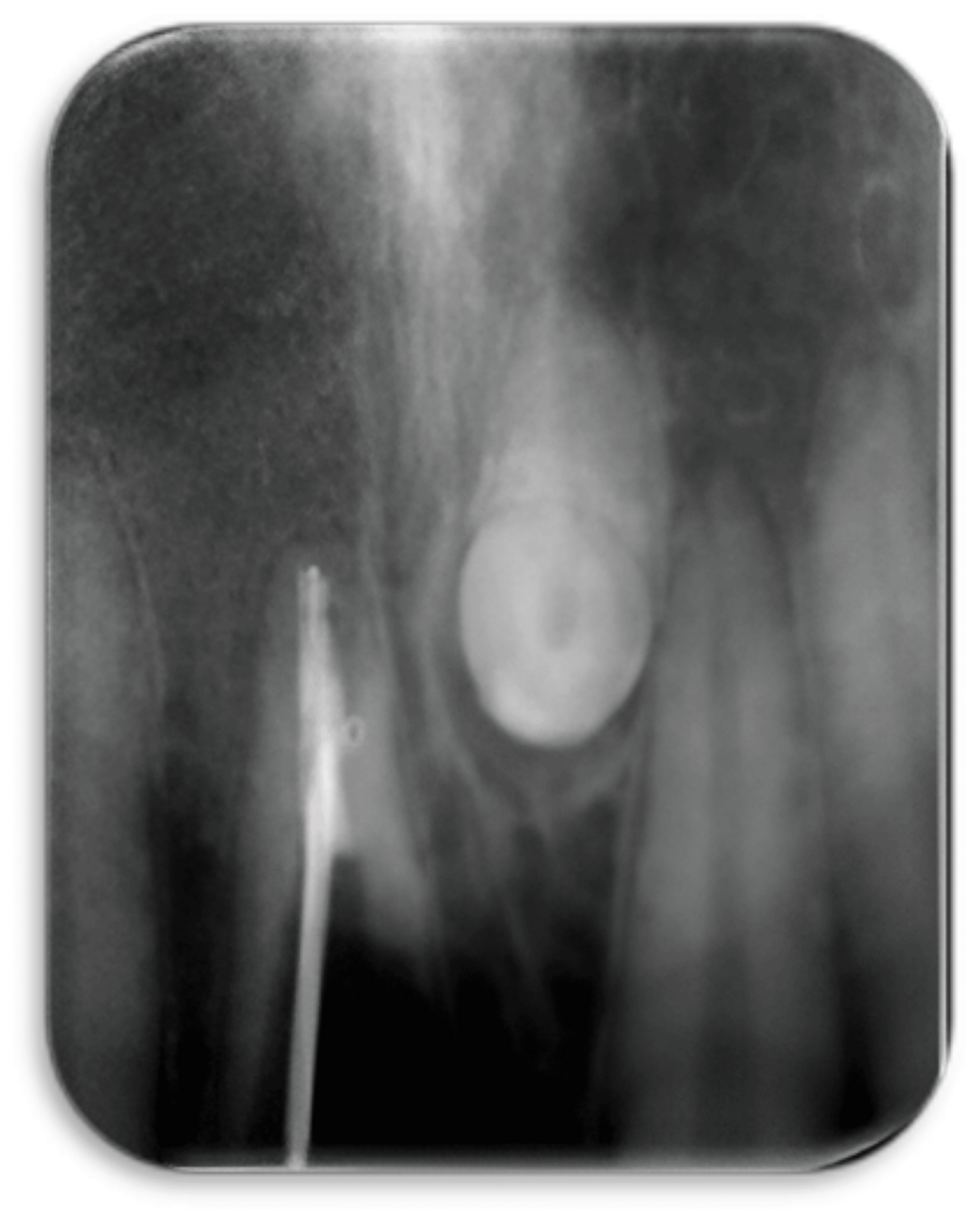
Case 2: RVG taken intra-operatively to assess the level of root resection RVG: Radiovisiography, digital x-ray.
